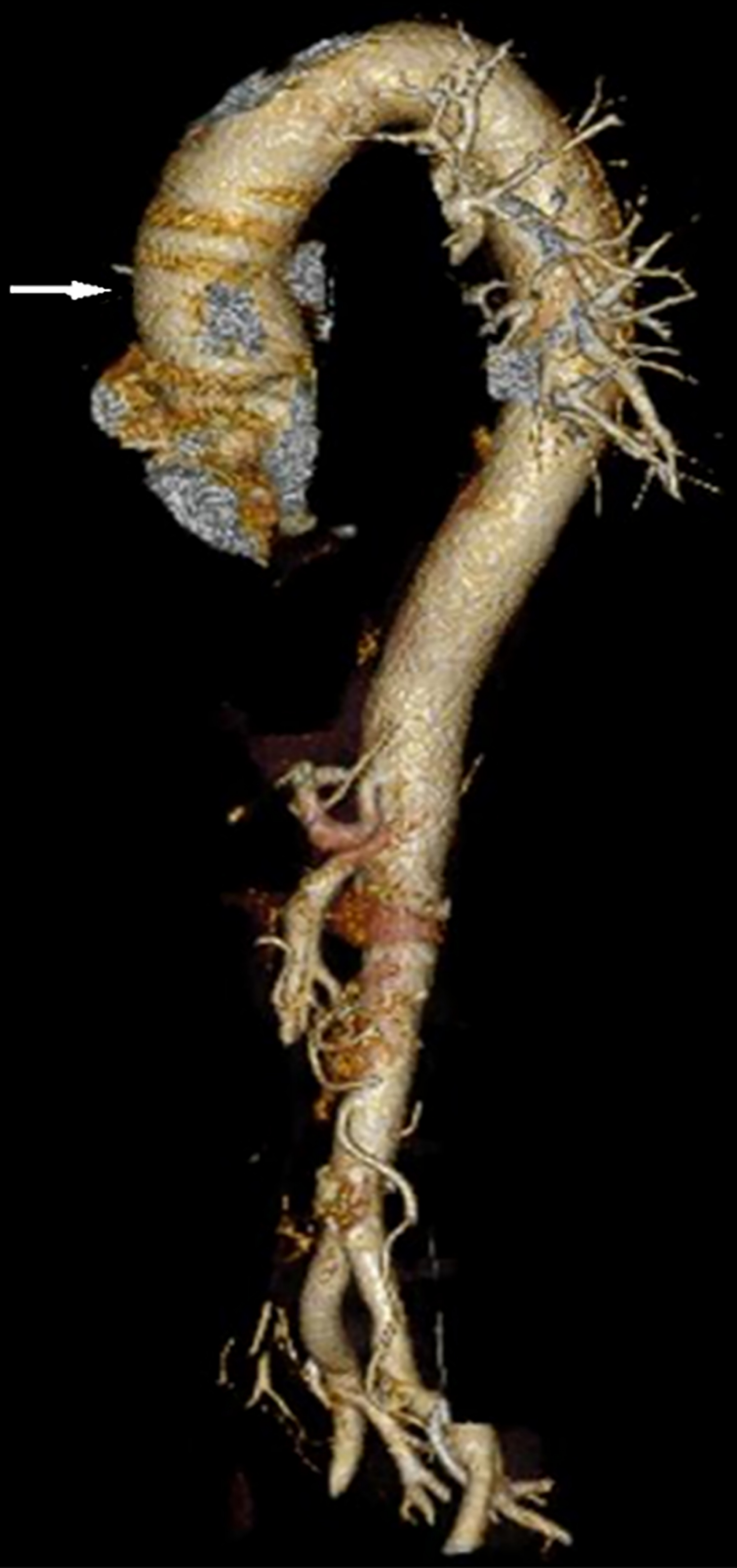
3D reconstruction of CT aortogram showing ectatic ascending aorta (white arrow).
